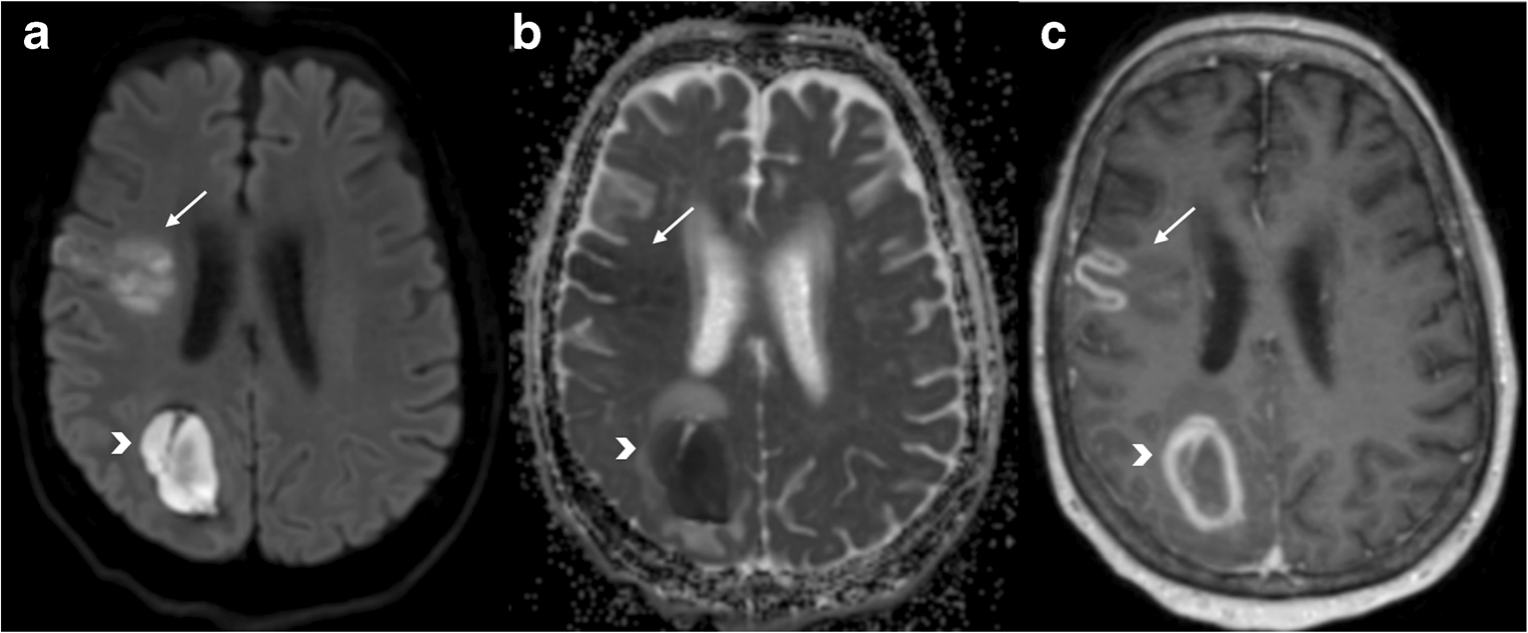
Subacute haematoma. Right parietal mass (arrowhead) showing diffusion restriction within the core on DWI and ADC maps (a and b, respectively) and a ring-enhancing pattern on T1 post gadolinium (c). This was a subacute haematoma. Clinical context is important to differentiate haemorrhage from abscess. There is also a subacute ischaemic lesion in the inferior right frontal lobe (arrows) that shows early pseudonormalisation of the ADC and gyriform enhancement post gadolinium
